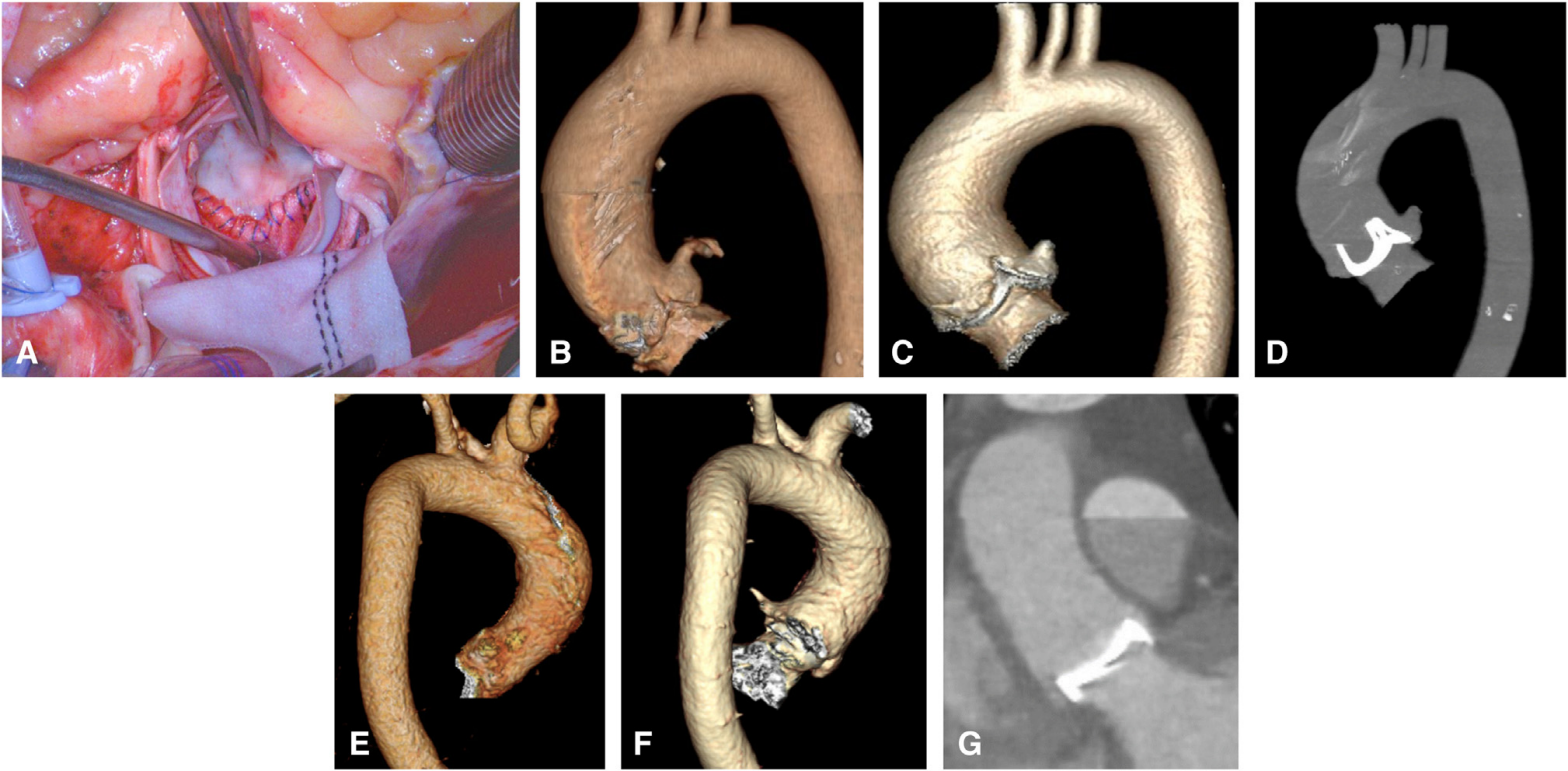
A, A large patch preserved the normal-sized basal ring and allowed a large prosthesis to be implanted, upsizing from a size 23 to size 29. B through D, The surgical aortic annulus was enlarged from 23 mm to a size 29 Magna Ease valve (Edwards Lifesciences). B, Preoperative computed tomography (*CT*) aortogram with 3-dimensional (*3D*) reconstruction. C, Postoperative CT aortogram with 3D reconstruction. D, Postoperative CT aortogram showed the bioprosthetic valve seated well without tilting, and the root and sinotubular junction were enlarged adequately for easy access for coronary artery catheterization and future valve-in-valve transcatheter aortic valve replacement. E through G, The surgical aortic annulus was upsized from 19 mm to size 27 Top Hat (Carbomedics) mechanical valve in a female patient with a body mass index of 53. E, Preoperative CT aortogram with 3D reconstruction, posterior view. F, Postoperative CT aortogram with 3D reconstruction, posterior view showing the Hemashield patch (Getinge). G, Postoperative CT aortogram showed the mechanical valve seated well without tilting.
